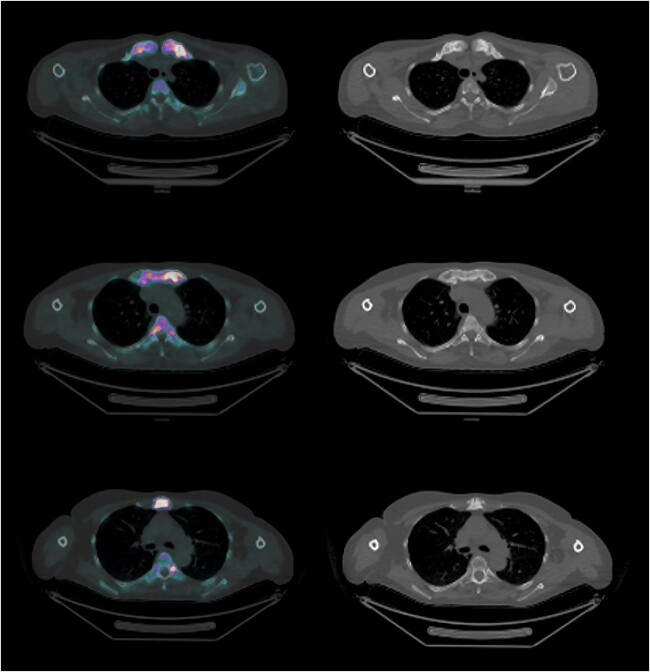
Transversal views of CNO on [^18^F]NaF-PET/CT visualizing clavicular (upper), costosternal (middle), and manubrial (lower) sclerosis, hyperostosis, and increased [^18^F]NaF-uptake. Abbreviations: CNO, chronic nonbacterial osteitis; [18F]NaF-PET/CT, sodium fluoride-18 positron emission tomography/computed tomography.
